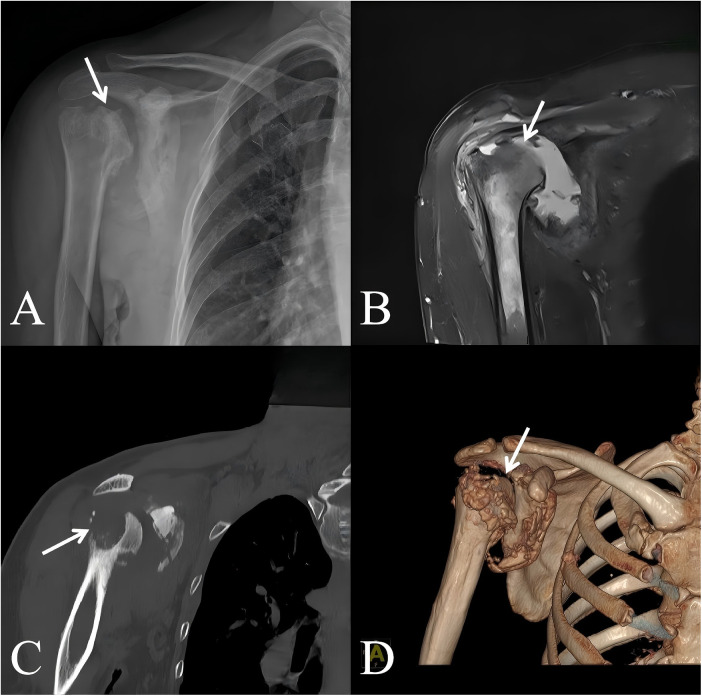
Preoperative right shoulder anteroposterior X-ray (A), MRI coronal view (B), CT coronal view (C), and 3D reconstruction (D). The white arrows in A–D indicate the osteolytic destruction of the humeral head.
